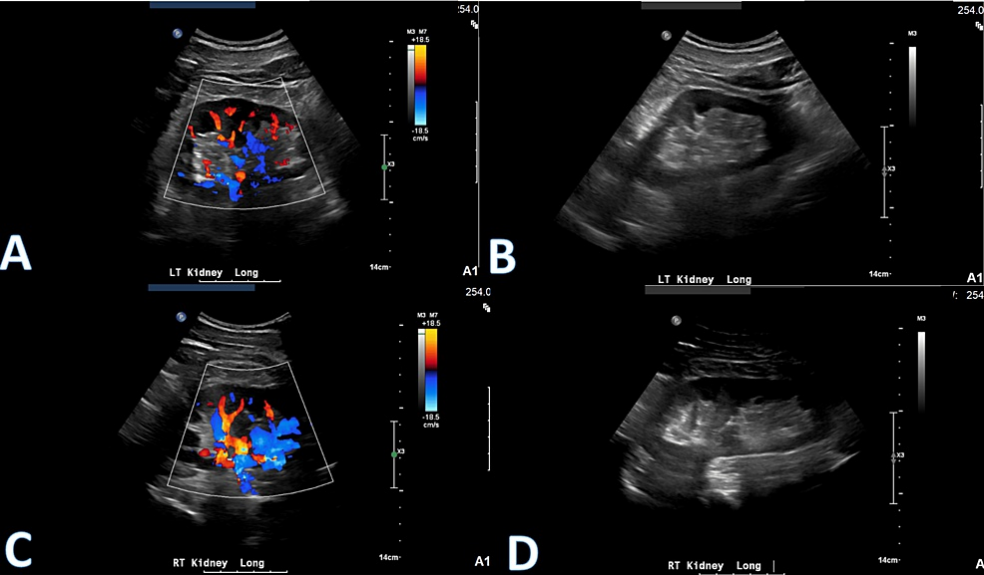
Bilateral renal artery Doppler ultrasound showing no significant findings
